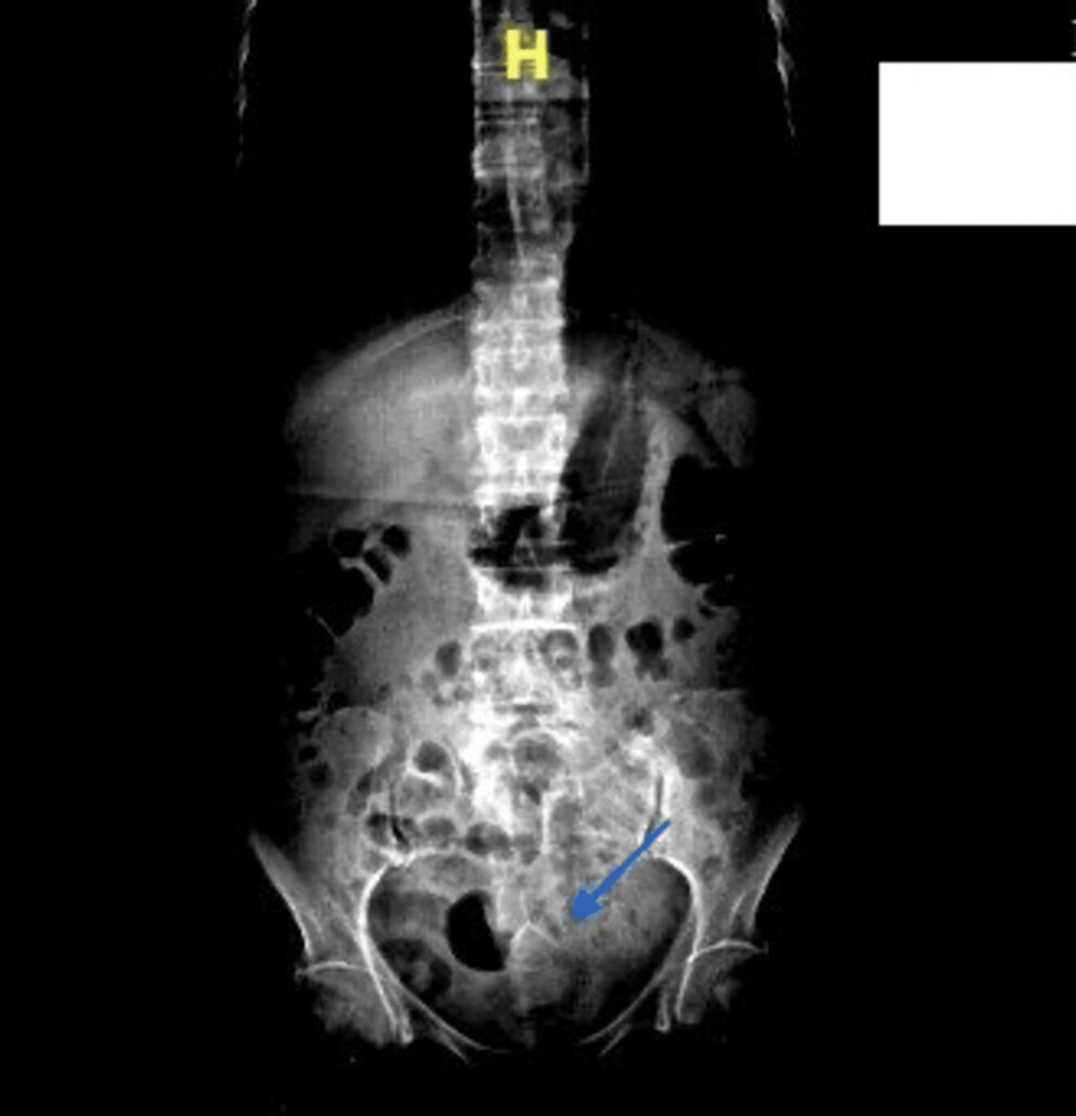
Plain radiograph of the abdomen on presentation showing a T-shaped foreign body.
